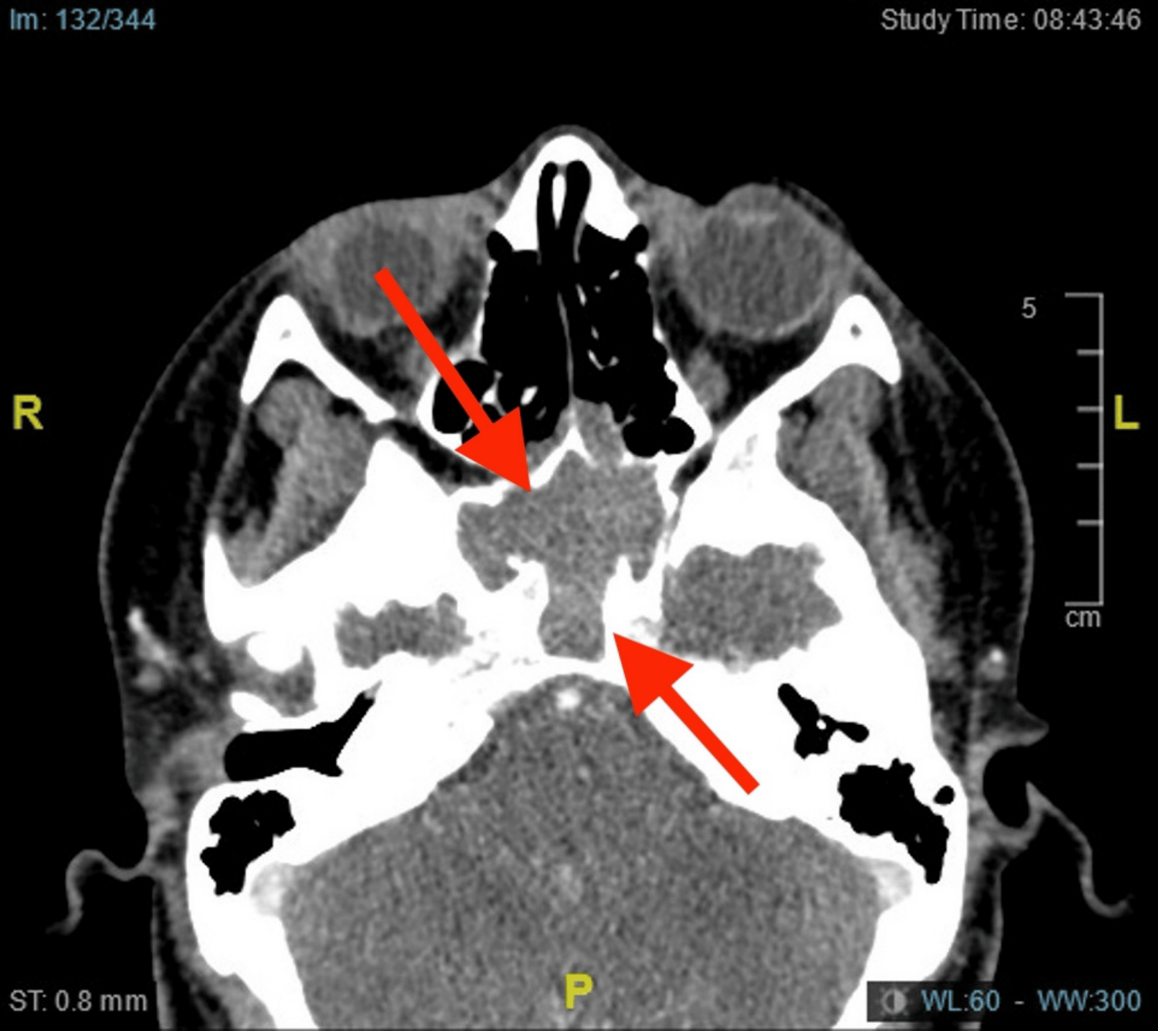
In the CT scan of the paranasal sinuses, hypodensity of soft tissue was identified, with occupation of the sphenoid sinus extending to the ostium of the sphenoid sinus, associated with bone erosion and partial destruction of the clivus (red arrow).
